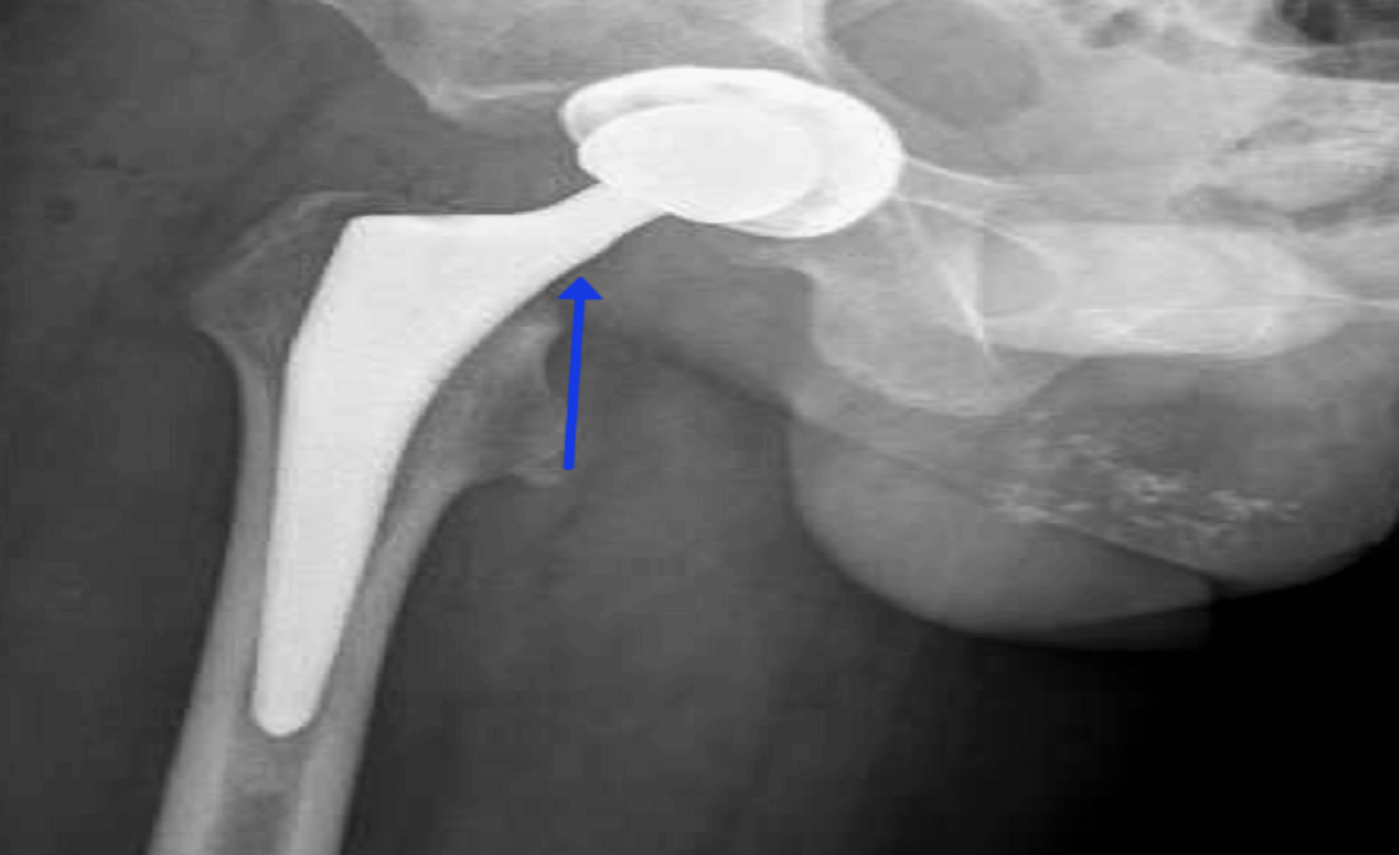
Postoperative X-ray showing good positioning of the implant
